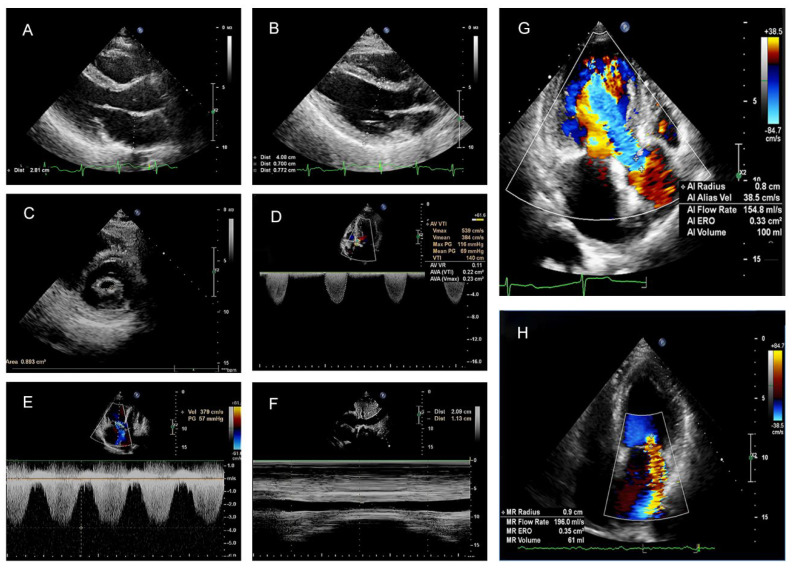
Representation of measured values based on echocardiographic views. (A) The measurements of the left atrial in parasternal left ventricular long axis (PLAX) view. (B) The measurements of the interventricular septum (IVS), left ventricular posterior wall (LVPW), and left ventricular end-diastolic diameter (LVEDD) in PLAX view. (C) Measurement of the mitral valve area in a patient with rheumatic heart disease; the mitral valve area was manually outlined and the mitral valve area is 0.89 cm^2^. (D) The aortic valve area was calculated using continuity equations, and the effective valvar orifice area (EOA) of the aortic valve is 0.23 cm^2^. (E) A continuous wave Doppler was used to measuring the end-expiration peak velocity of the tricuspid regurgitant (TR) jet. (F) The IVC size was measured in M-mode at the subcostal window. The IVC—CI (inferior vena cava collapsibility index) was calculated as follows: IVC-CI = [(IVC diameter in expiration—ICV diameter in inspiration)/ (IVC diameter in expiration)] × 100. Right atrial pressure (RAP) was estimated from the IVC-CI. (G) Quantitative assessment of aortic regurgitation using the proximal isovelosity surface area method (PISA). The effective regurgitant orifice area (EROA) is 0.33 cm^2^ and the regurgitant volume (RV) is 100 mL. (H) Quantitative assessment of mitral regurgitation by PISA. The EROA is 0.25 cm^2^ and the RV is 61 mL.
